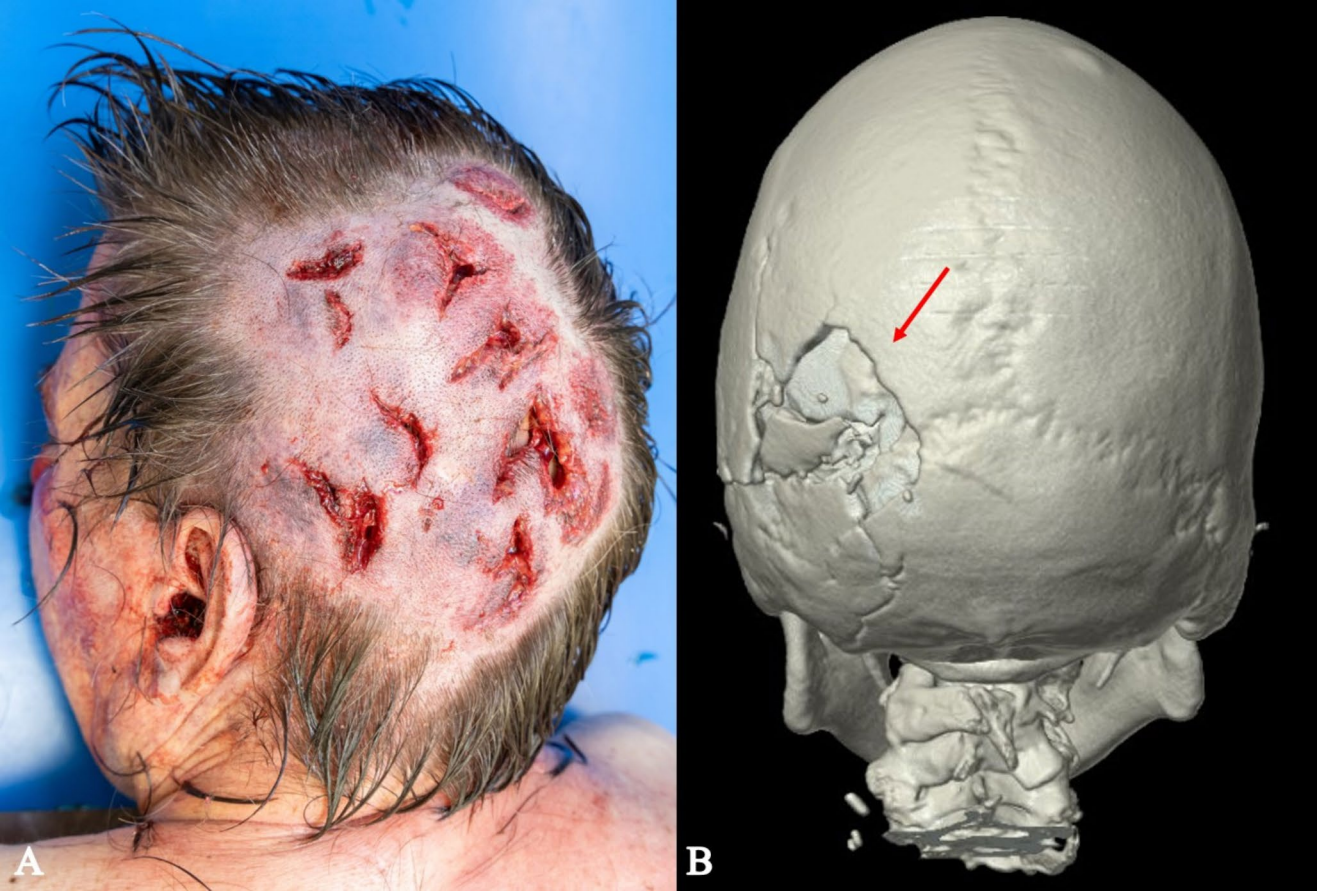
Multiple hammer blunt force injuries on the scalp (A) and CT 3d reconstruction of the depressed fracture complex in the right occipital region (red arrow) (B)
